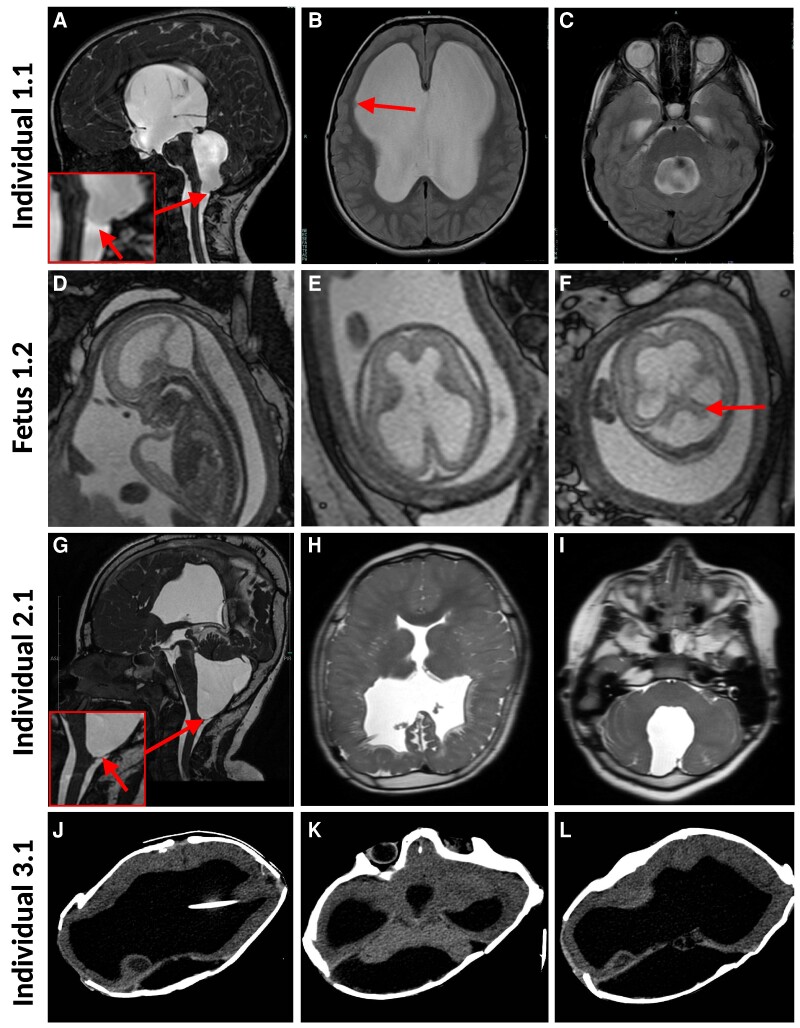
Brain images of four individuals with a loss of *PLAT* function. (A–C). MRI images from Individual 1.1 at 7 years of age. (A) Sagittal constructive interference in steady state (CISS) image. The arrow points to CSF flow artefacts (enlarged in the inset) suggesting the presence of a membrane (not visualized because of these artefacts) at the outlets of the fourth ventricle. (B) Axial T2 image showing significant enlargement of both lateral ventricles with frontal predominance and absent septum pellucidum and subependymal heterotopia (arrow). (C) Axial T2 image showing dilated 4th ventricle with turbulent cerebrospinal fluid. (D–F) Images from foetus 1.2 at 23 3/7 weeks of gestation. (D) Sagittal T2 image showing dilated fourth ventricle. (E). Axial T2 image showing dilatation of both lateral ventricles. (F). Oblique coronal image showing hypoplastic cerebellum (arrow) and dilated fourth ventricle. (G–I). MRI images from Individual 2.2 at 19 months of age. (G). Sagittal 3D Fiesta showing a membrane at the central outlet of the fourth ventricle (arrow). (H). Axial T2 reformat showing absent septum pellucidum, subependymal heterotopia and ventricular shunt with residual hydrocephalus. (I). Enlargement of fourth ventricle with dysplastic cerebellar hemispheres. (J–L). Axial CT-scan images from Individual 3.1 at 1 year of age, showing significant enlargement of both lateral ventricles (J, K) and the fourth ventricle (L).
